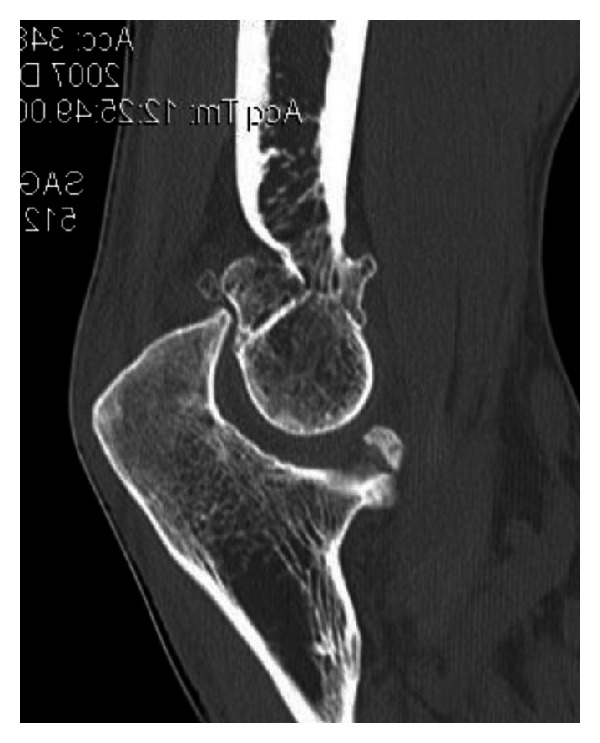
Sagittal CT image demonstrating osteophytes within the olecranon and coronoid fossae of the distal humerus.
